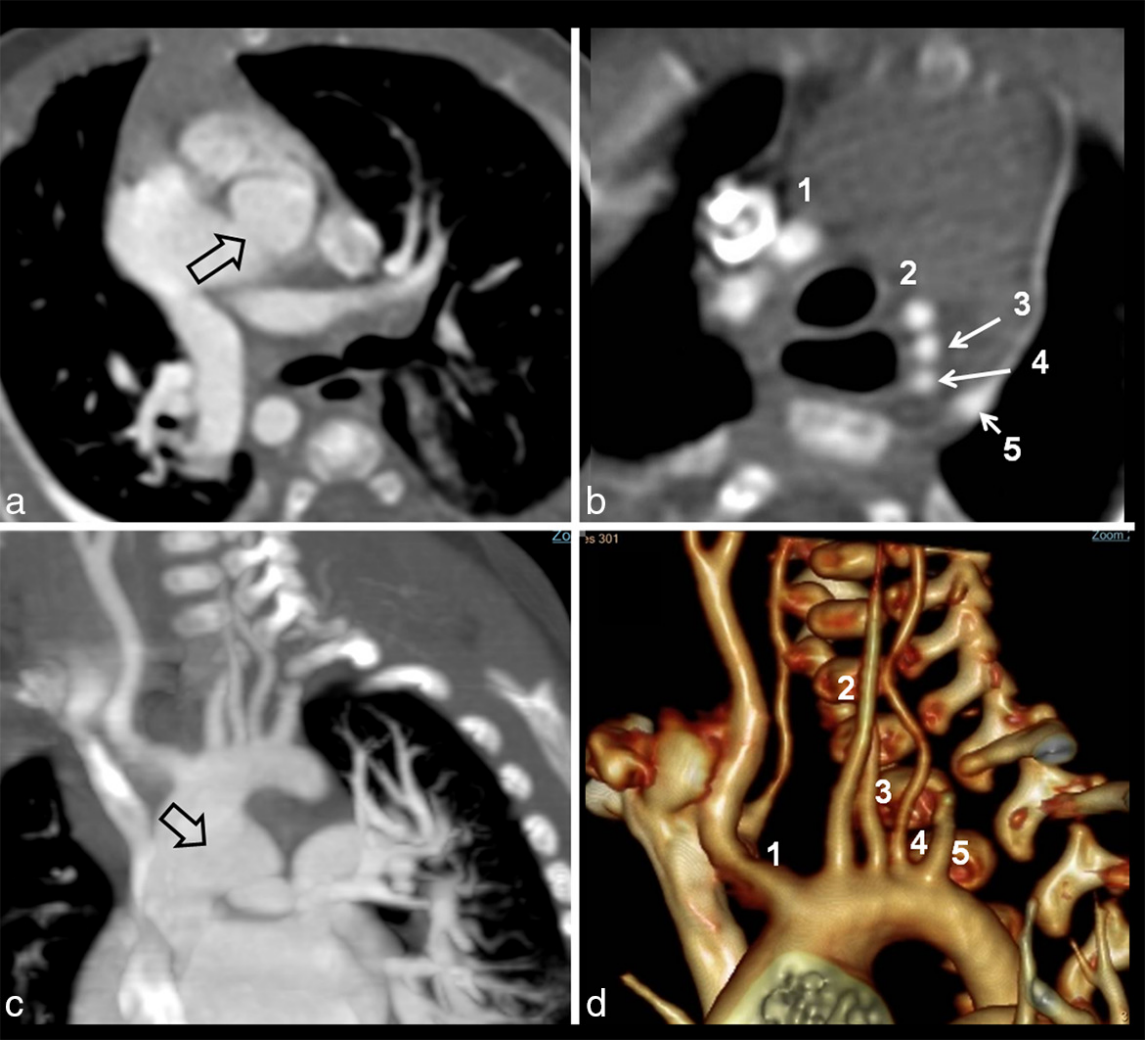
(a) Axial CT image at the level of main pulmonary artery shows an APW (open arrow). Axial image above the level of AA (b) shows six arteries, five directly arising from the aorta (1, innominate artery; 2, LECA; 3, left ICA; 4, left VA; and 5, LSA). Coronal maximum intensity projection image (c) demonstrates an APW (open arrow) and five arteries arising from the AA. Three-dimensional surface rendered view (d) showing the branches of AA (1–5). AA, aortic arch; APW, aortopulmonary window; ICA, internal carotid artery; LECA, left external carotid artery; LSA, left subclavian artery; VA, vertebral artery.
